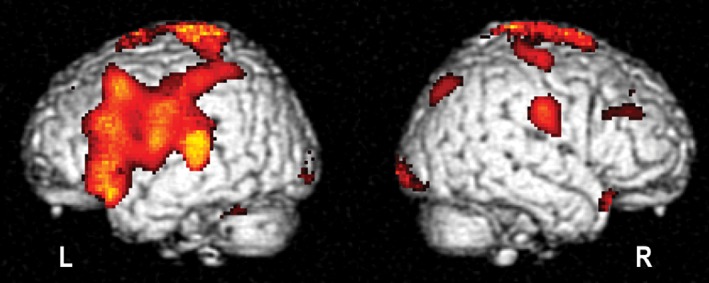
99mTc – hexylmethylpropylene amineoxine – single‐photon emission computed tomography scan of subject's cerebral perfusion compared against age‐matched controls, rendered on template brain at *P* < 0.01 threshold. Scan shows predominant left dorsolateral prefrontal cortex and insula hypoperfusion consistent with a non‐fluent aphasic syndrome.
